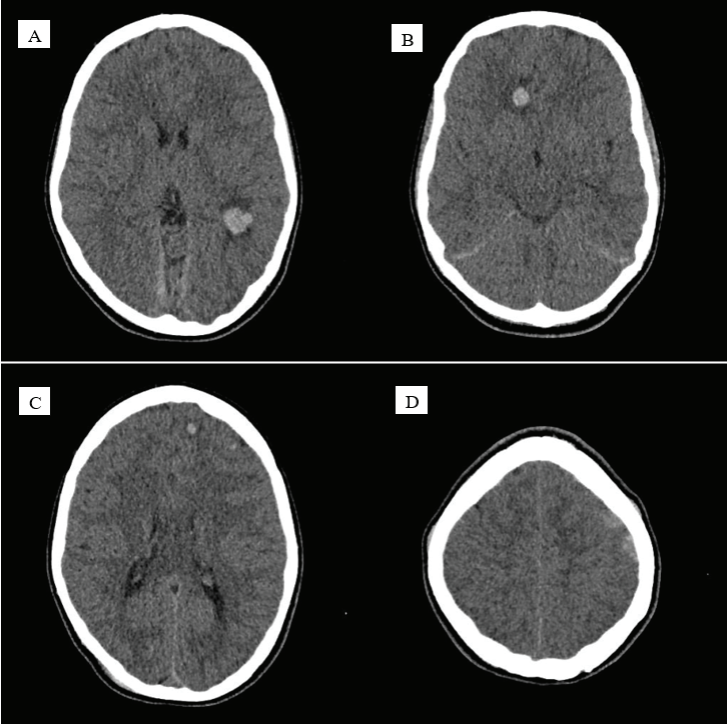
Head CT of Case 2 at admission showing multiple intracerebral haemorrhages in the left temporal-parietal lobe (A) and in both frontal lobes (B, C). Subdural hematoma in left parietal lobe is shown in picture D.
